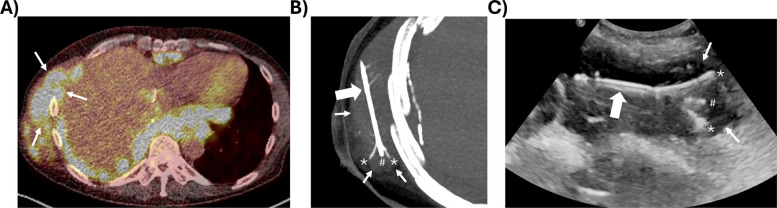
CT, US and PET of target tumor and multi-tine injection needle. A PET-CT fusion demarcates the tumor tissue (thin arrows) providing a target for drug delivery. B CT maximum intensity projection image verifies the position of the base needle (large arrow) and its tip (crosshatch) and 2 of 3 deployed injection tines (asterisks) within the tumor. Tines can be extended to enable 1 cm incremental deployments up to an array diameter up to 5 cm. To deliver drug to more tissue, the tines were partially retracted during injection. With full retraction, the needle could be rotated with redeployment and injection. C Ultrasound enabled real-time imaging of needle and tine location in relation to the fused US and CT and could also identify extravasation or extra-tumor leakage of the injectate
